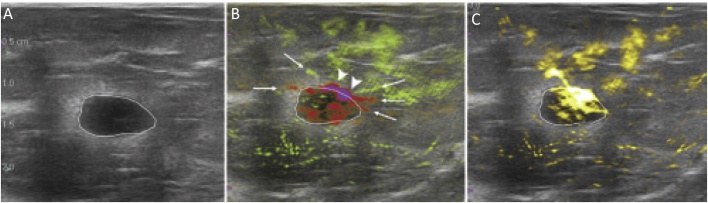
From the Maestro study using the Seno Imagio linear system: a grade II invasive ductal carcinoma that was upgraded from PA/US findings. (A) Photoacoustic US shows findings similar to those from conventional US leading to BI-RADS 4A classification. (B) PA/US shows multiple internal vasculature including deoxygenated (red) vessels with intense deoxygenated anterior boundary zone blush (arrowheads) and boundary zone neovessels (arrows). (C) PA/US total hemoglobin map shows markedly increased hemoglobin within the central tumor. The mass was upgraded from BI-RADS 4A from gray-scale US and US Doppler, to BI-RADS 4C from PA/US. (Reproduced with permission of the authors and publisher.)
